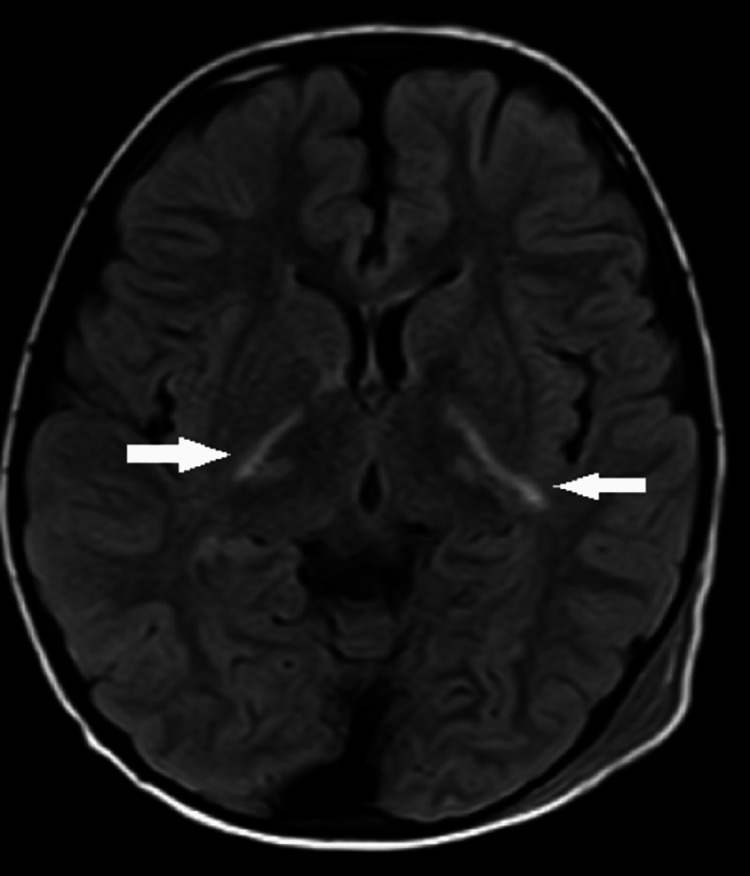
MRI brain axial FLAIR images depicting hyperintensities involving bilateral posterior limbs of internal capsule FLAIR: fluid-attenuated inversion recovery
